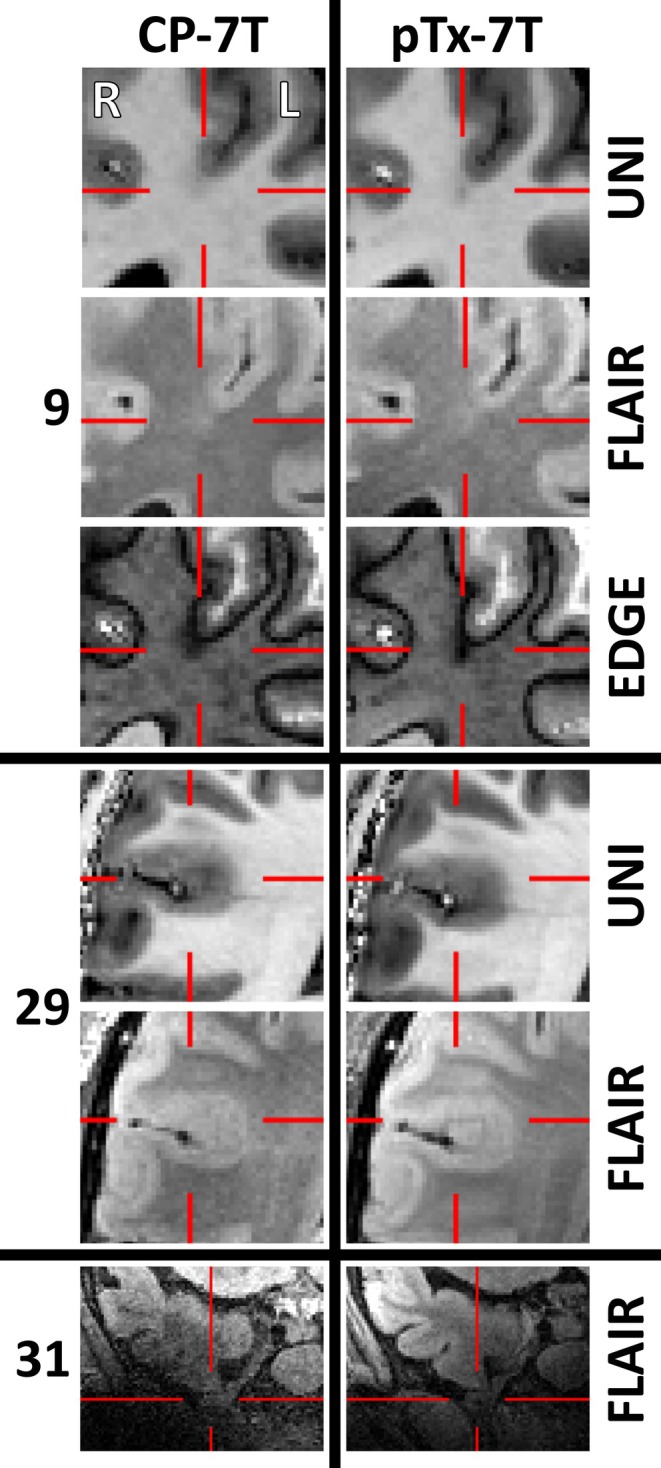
Further lesions better visualized with pTx than CP acquisitions. Patient 9: Focal cortical dysplasia much more prominently displayed and crisply defined, especially in the EDGE contrast. Patient 29: Focal cortical dysplasia not immediately evident on CP images, but clearly demonstrated with pTx. Patient 31: Inferior temporal encephalocele falling in an area of high signal dropout and low signal‐to‐noise ratio in CP FLAIR. Although the encephalocele can be seen with both acquisition methods it is impossible to assess the integrity of surrounding cortex in the CP images. MDT discussions often focus on whether an encephalocele may be associated with a more widespread cortical lesion and a more extensive resection may be warranted in addition to the skull‐base repair. This could be conducted with much greater confidence based on the pTx images compared to the CP images.
